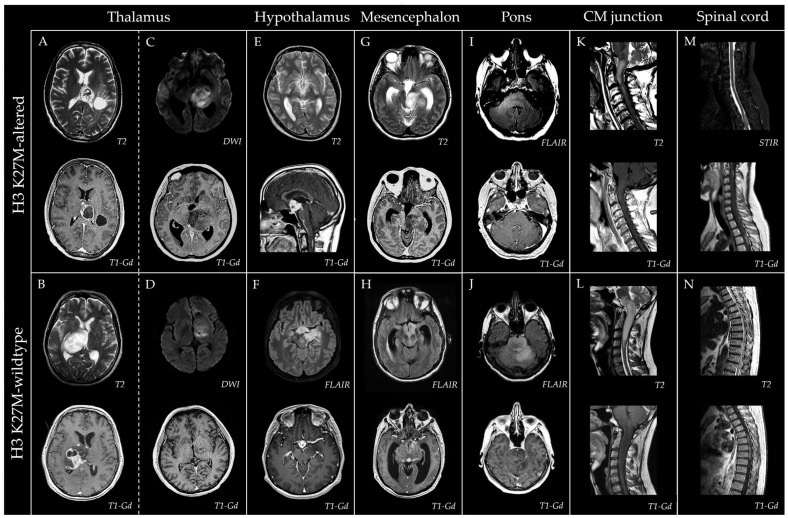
Representative MRI images of adult midline diffuse gliomas. Thalamic gliomas with variable amount and pattern of gadolinium contrast enhancement, including vivid ring-like (A), mixed ring-like and solid (B), minute nodular (C), and minute ring-like enhancement (D). Variable extent and intensity of focal restricted diffusion was common (C,D). A vividly enhancing hypothalamic metastasis of a H3 K27-altered DMG with an initial lumbar spinal presentation, with apparent leptomeningeal enhancement encompassing the brainstem (E). Hypothalamic involvement was frequent in H3 K27 wildtype gliomas, here with a contrast-enhancing core and a non-enhancing infiltrative extension to adjacent structures (F). Severe mesencephalic involvement in a H3 K27-altered DMG with minimal focal contrast enhancement, leading to hydrocephalus due to cerebral aqueduct compression (G). A multifocal H3 K27 wildtype hypothalamic glioma diffusely infiltrating the mesencephalon, similarly leading to hydrocephalus due to cerebral aqueduct obstruction by a solid contrast enhancing component (H). Diffusely infiltrating H3 K27-altered DMG involving the pons and extending toward the cerebellum without contrast enhancement (I). A strikingly similar pattern in a H3 K27 wildtype case (J). Extensive H3 K27-altered DMG with an infiltrative and non-enhancing pattern involving the medulla oblongata and the cervical spinal cord (K) and a similar growth pattern in a wildtype glioma (L). Multiple diffusely enhancing, solid, leptomeningeal drop metastases in the cervical and thoracic spinal cord of a H3 K27M-altered DMG initially presenting in the lumbar spinal cord (M). Focally enhancing H3 K27M wildtype diffuse glioma expanding through the entire thoracolumbar spinal cord, which eventually reached the pons (N).
